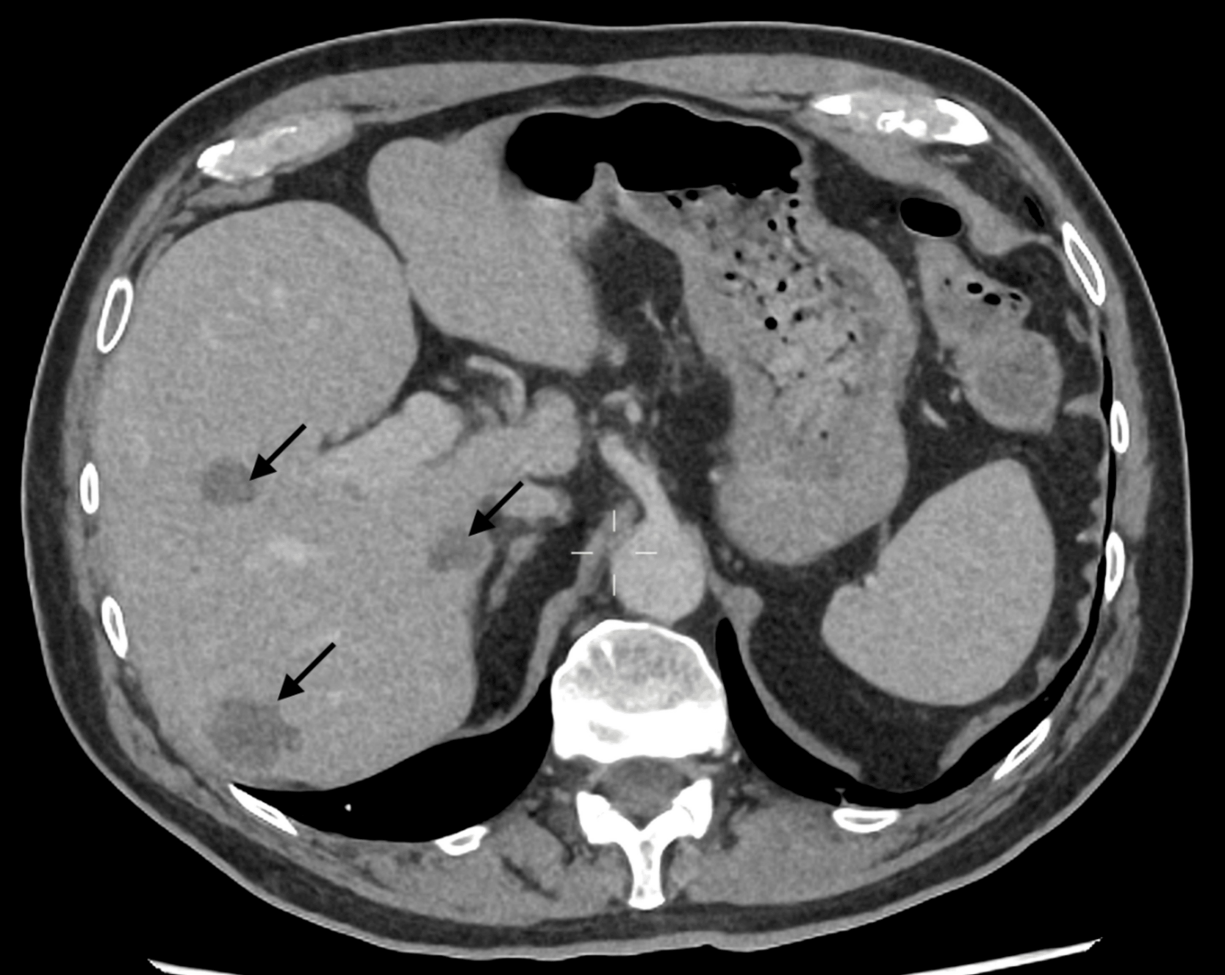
CT of the abdomen showing multiple hypoattenuating hepatic abscesses (arrows).
